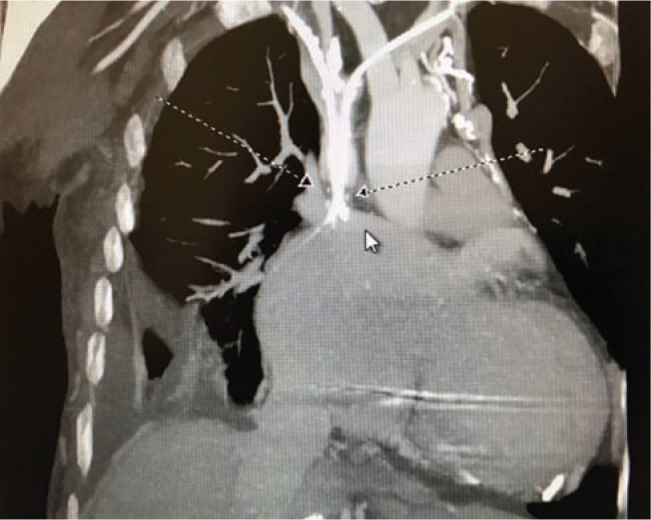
Postoperative computed tomography angiogram shows complete occlusion of the superior vena cava just above the right atrium (arrows).
